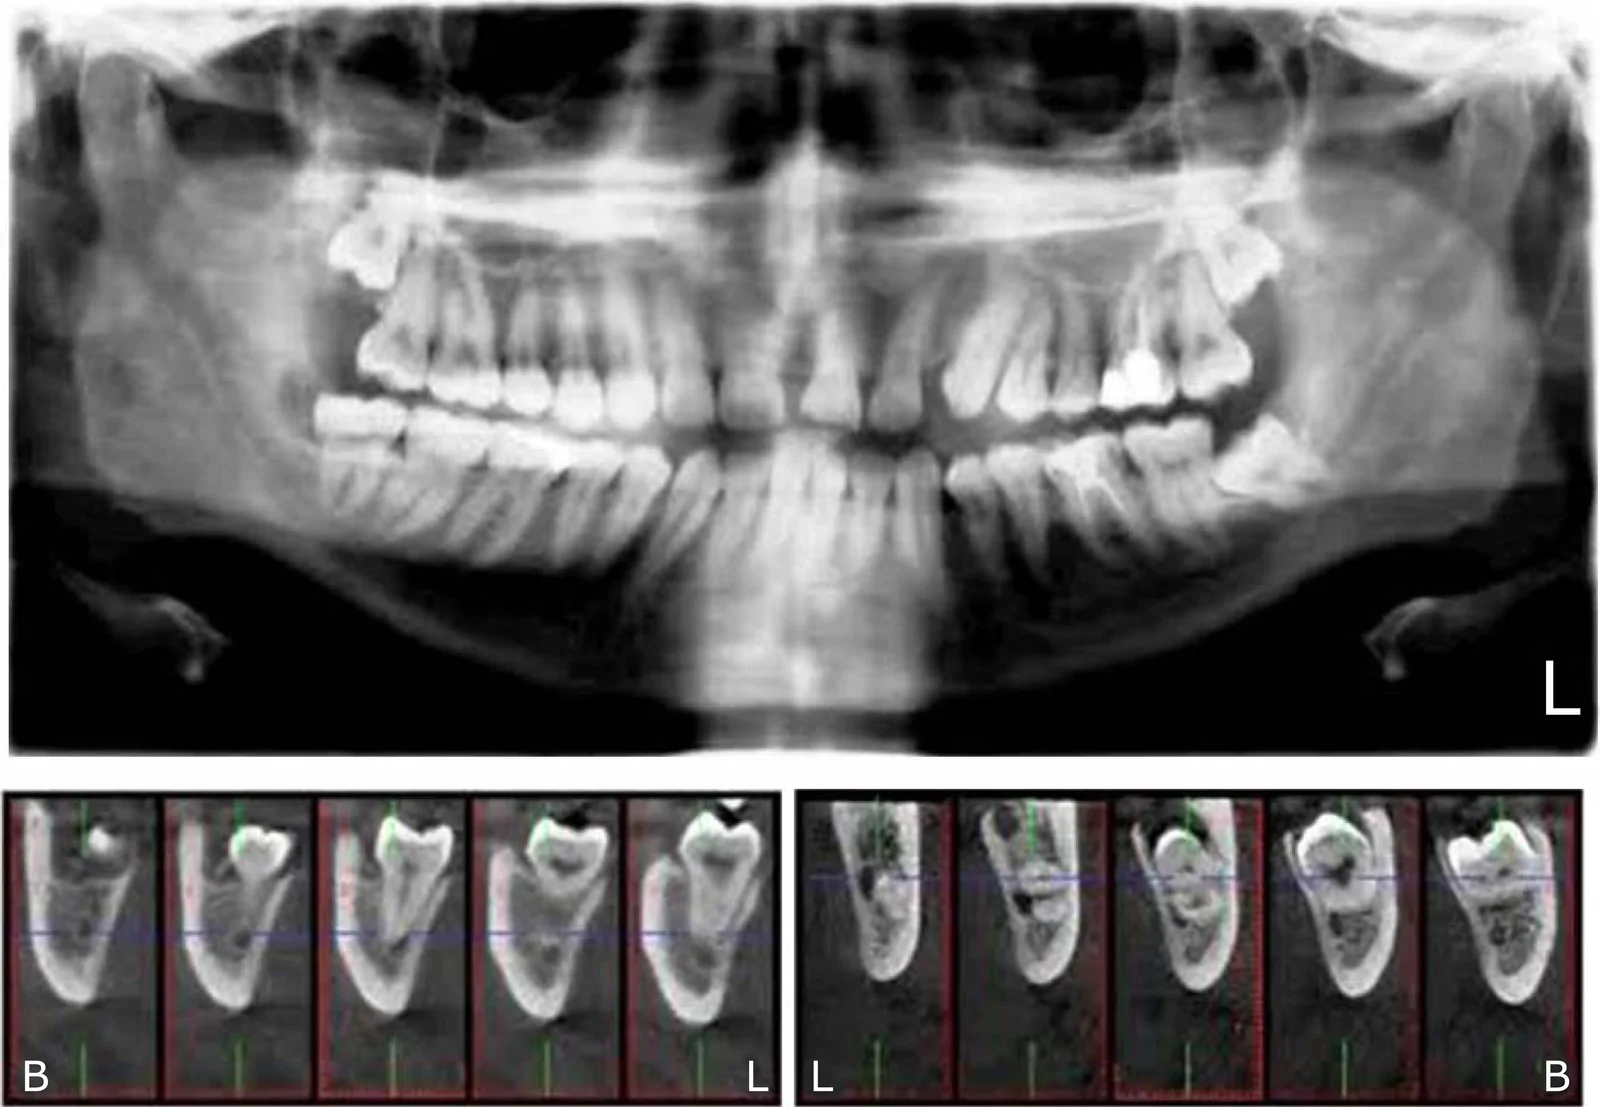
Comparison of OPG and CBCT images for assessment of cortication.
